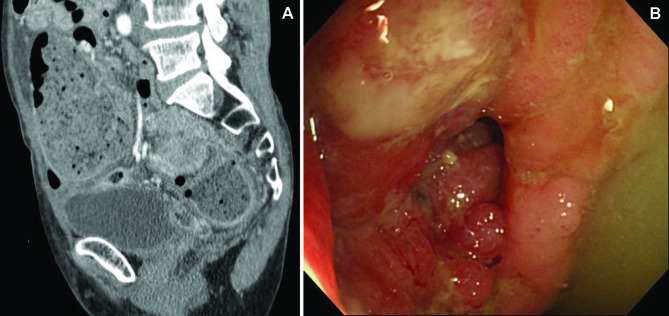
(A) Abdominal CT showing sigmoid carcinoma and the dilated colon; (B) Sigmoid carcinoma showing the obstructed lumen which can be detected.
